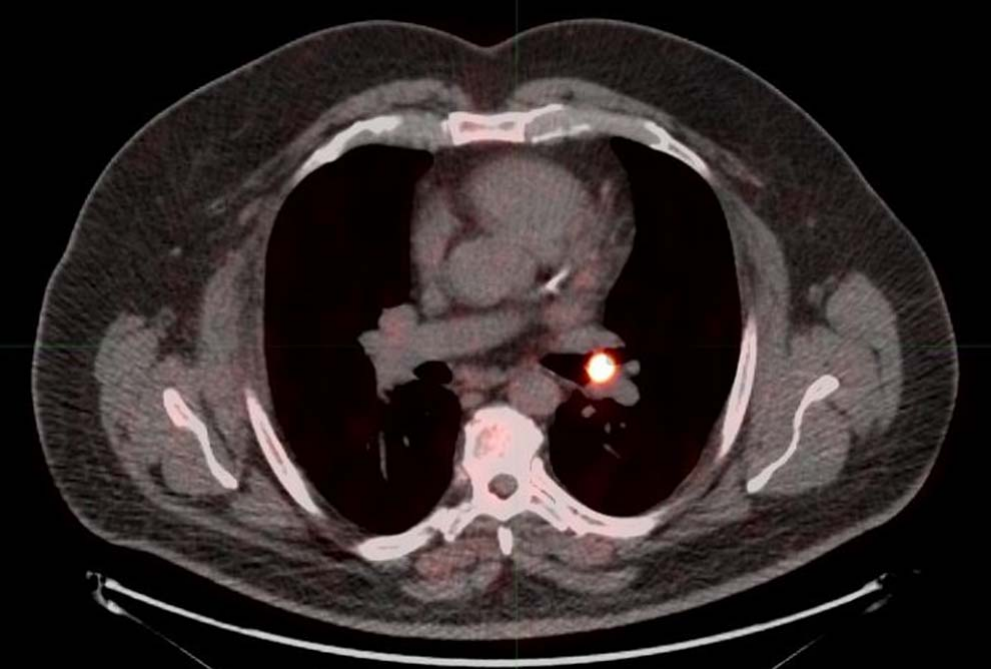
Fused gallium-68 DOTATATE positron emission tomography-computed tomography axial image demonstrates focal increased somatostatin receptor avidity within the left upper lobe endobronchial mass consistent with a neuroendocrine tumor.
